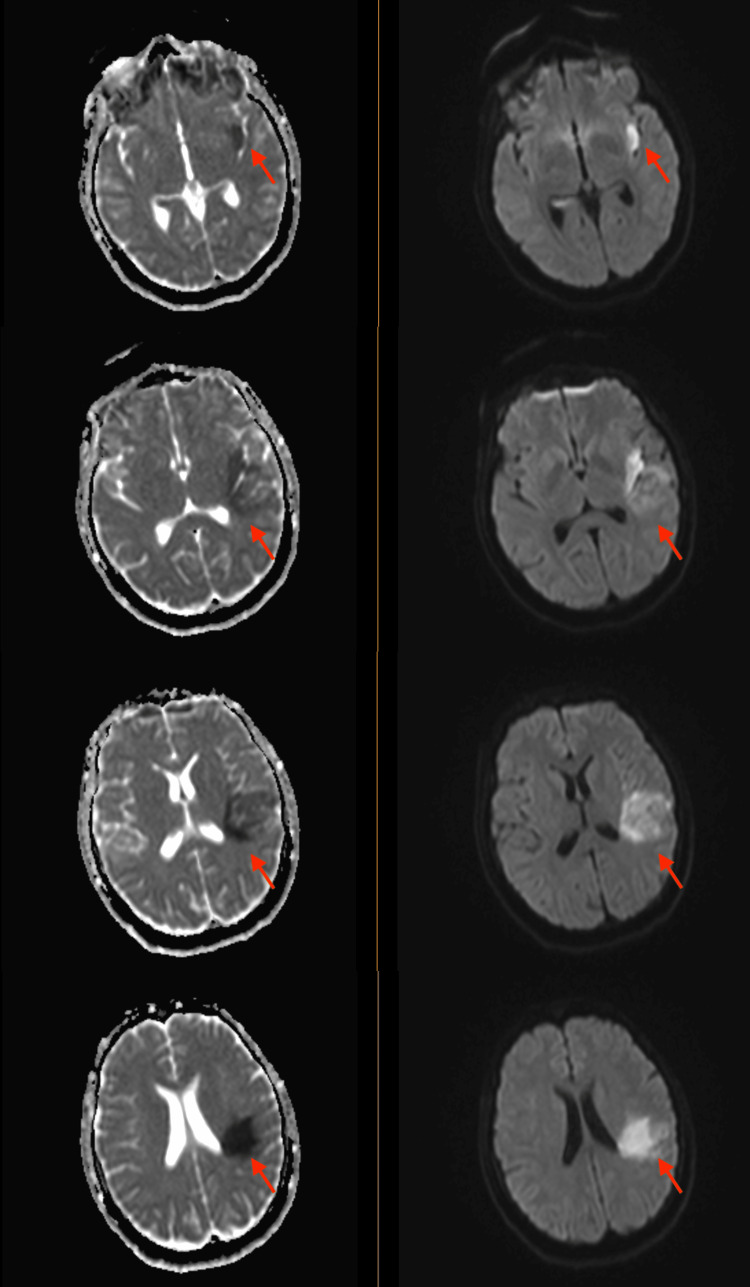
MRI of the brain showing early subacute ischemia in the left insula posteriorly and frontoparietal lobe in the middle cerebral artery territory, without any signs of hemorrhage, midline shift or cerebral edema.
